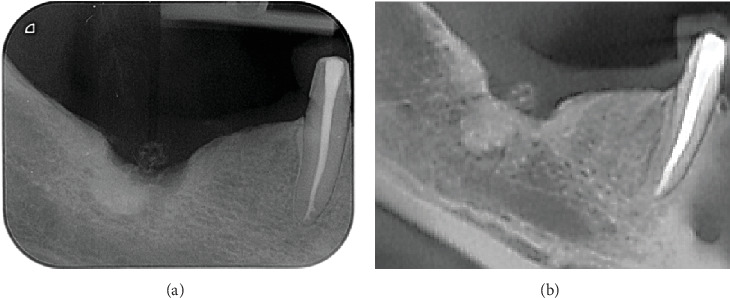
(a) Preoperative intraoral periapical radiograph of the region of missing mandibular right molars and (b) a reconstructed panoramic CBCT image (cropped) through the same region, revealing a bony sequestrum, slight soft tissue swelling, and sclerotic area in the underlying bone.
